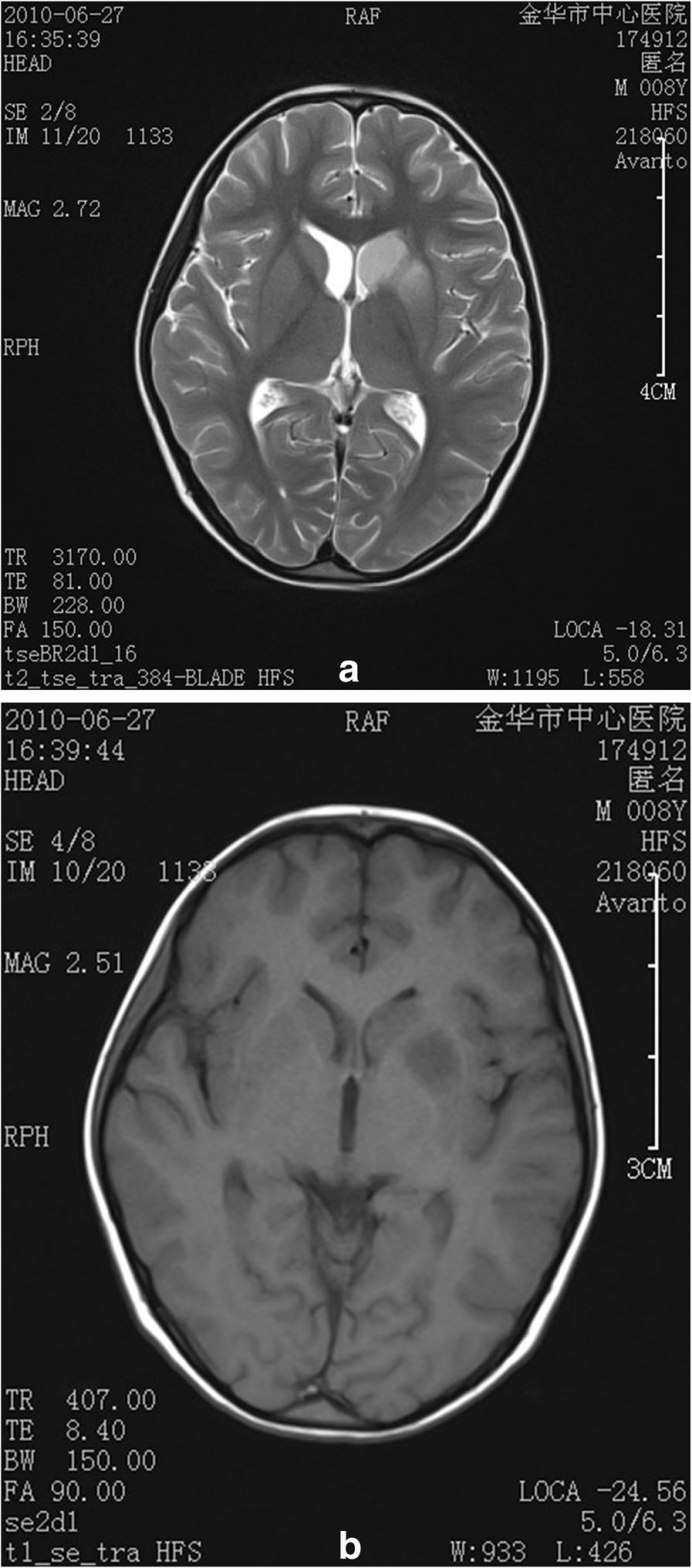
a-b Magnetic resonance imaging (MRI) of the brain showed a T2W1-weighted high-intensity signal (a) and a T1W1-weighted low-intensity signal of the left lentiform nucleus, caudate nucleus and temporal lobe (b), suggesting an acute infarction of the brain, including the above regions the first day after transfer
